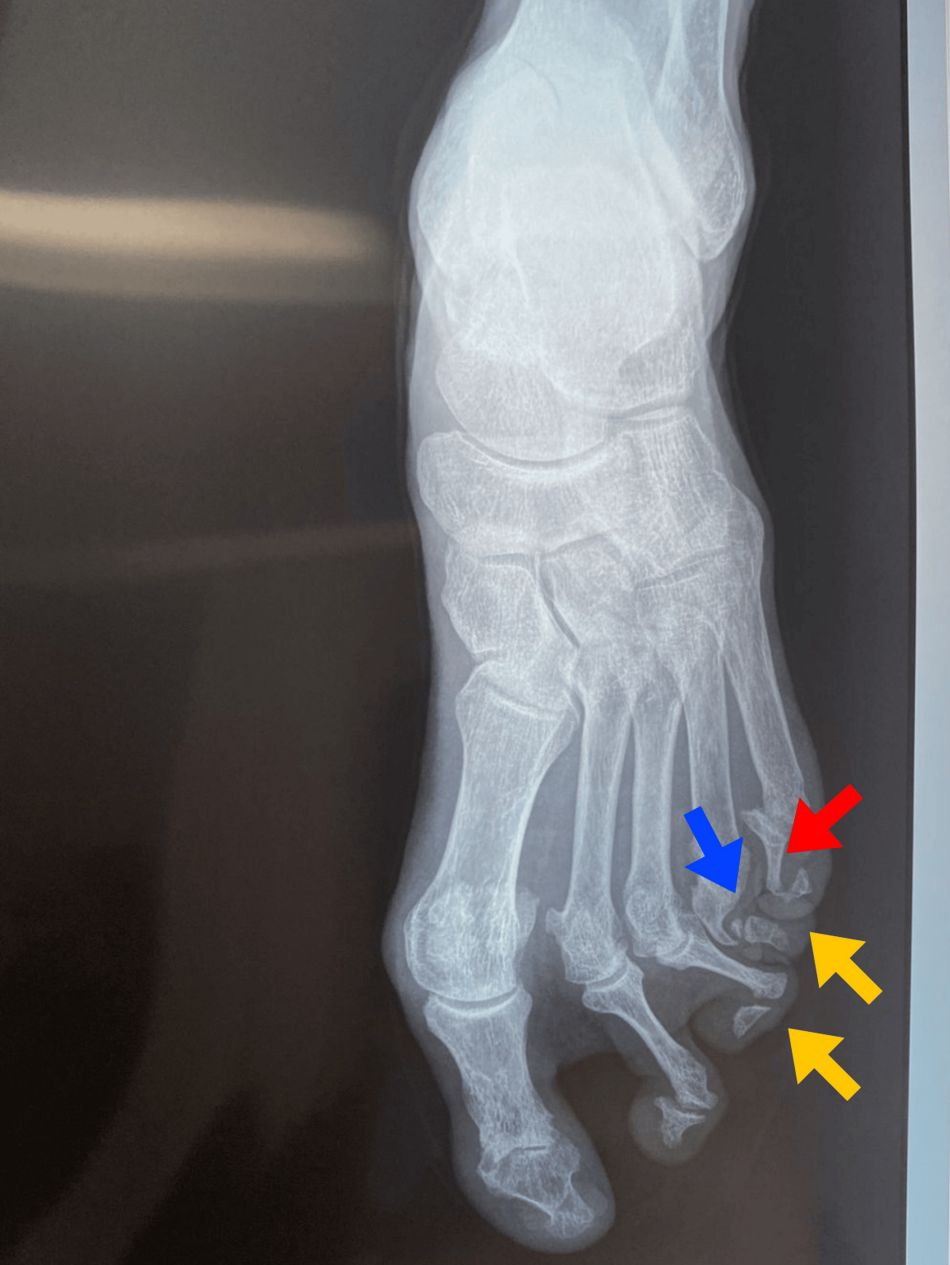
Radiograph showing osteolysis (yellow arrows), pencil-in-cup deformities (red arrow), and telescoping digits (blue arrow) in 2nd–5th toes. Note the sparing of thumb joints.
